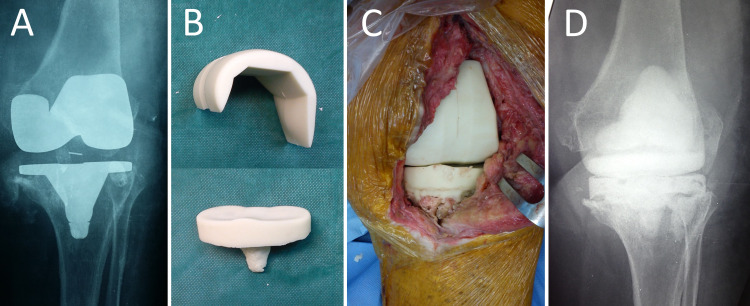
PJI of the left knee of a 69-year-old woman with Staphylococcus epidermidis-infected TKR. A. Preoperative radiograph of the left knee. B. The prefabricated articulated knee ALCS will be implanted after removal of the infected prostheses and extensive debridement and irrigation of the joint space. C. Intraoperative photograph after implantation of the spacer. D. Anteroposterior radiograph of the left knee after spacer implantation. PJI: prosthetic joint infection, ALCS: antibiotic-loaded cement spacer, TKR: total knee replacement.
